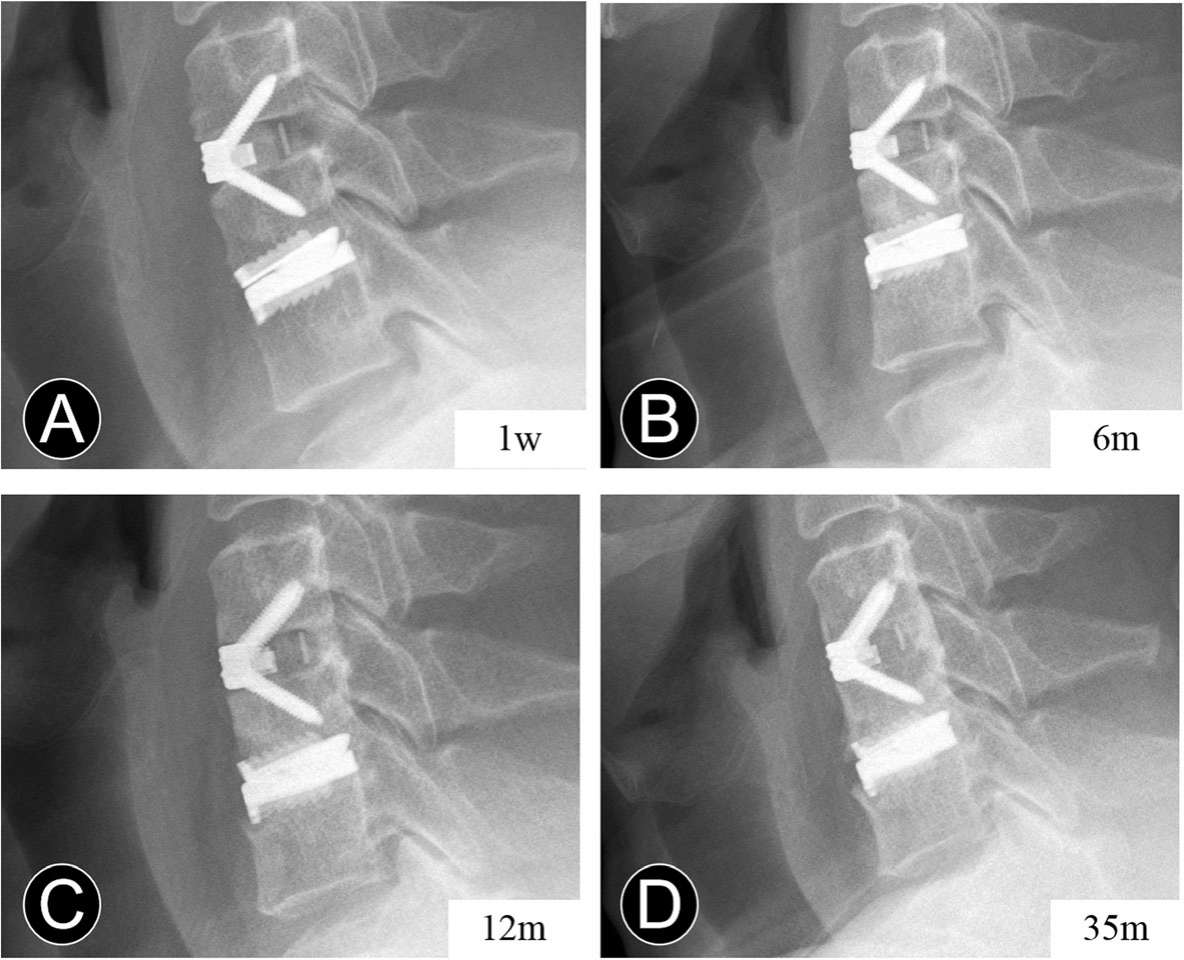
Serial postoperative radiographs of a 47-year-old man who underwent contiguous 2-level hybrid surgery at C5-C7. a and b The lateral radiographs show the anterior bone loss occurred at 6 months after surgery. c and d The 12-month and 35-month postoperative lateral radiographs show the anterior heterotopic ossification occurred after the non-progressive bone loss
